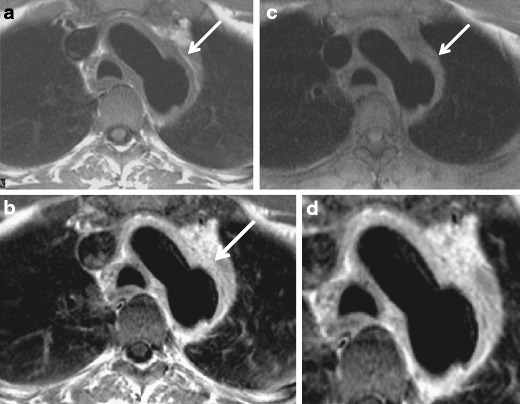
a–d A 51-year-old male with chest pain. Spin-echo T1-weighted (a), post-gadolinium enhanced (b) and proton density (c) images of the aortic arch show diffuse and relatively uniform thickening of the aortic arch with focal aneurysmal dilatation of the posterior aspect of the distal arch (*arrow*). Vivid gadolinium enhancement is uniform and diffuse (b), being even more vivid at the mid-outer portion of the aortic wall (d), corresponding to a “double ring” appearance
